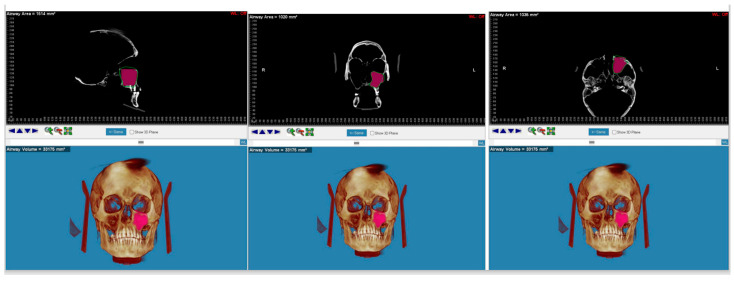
CBCT detection of maximum linear measurement along the sagittal axis, transversal axis, and vertical axis.
